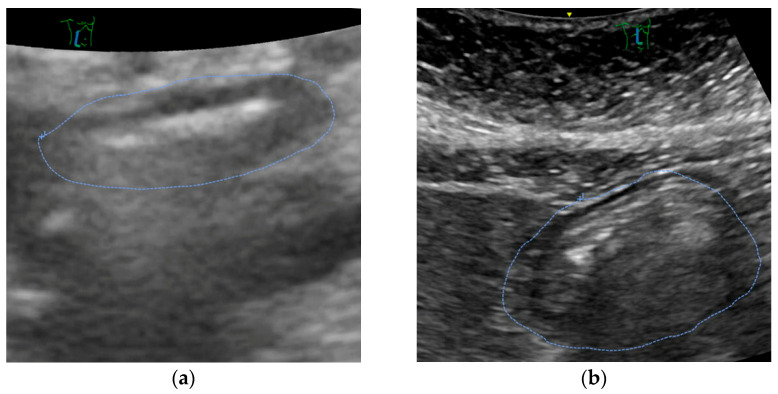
Screenshot of a representative gastric ultrasound exam performed in a patient (a) with an empty stomach (CSA 2.98 cm^−2^ corresponding to a calculated gastral volume of 70.5 mL) and (b) with a full stomach (CSA 12.0 cm^2^, 202 mL). Both patients had no liquids for more than two hours before the exam.
